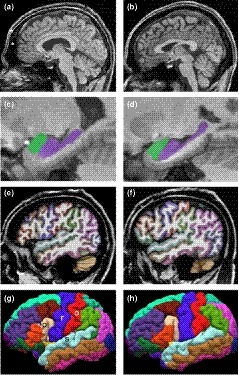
MRI findings. (a, c, e, g) The 26‐year‐old premutation carrier. (b, c, f, h) A 24‐year‐old male control. (a, b) A mid‐sagittal slice showing enlarged lateral ventricles and enlarged frontal subarachnoid spaces in the carrier (*). (c, d) Disproportionally enlarged hippocampal head the length of which is normally 35% of the whole length (Hackert et al., 2002).^23^ Hippocampus, purple; amygdala, lime. (e, f) A sagittal slice of the left hemisphere showing more complex folding in the carrier at the junction of the frontal, parietal, and temporal lobes. (g, h). A lateral view of the reconstructed cortex of the left hemisphere showing more complex folding in the pars opercularis (p, light brown, LGI = 4.49, *Z* = 2.38, 1.73%), precentral gyrus (r, purple, LGI = 3.79, *Z* = 4.32, 0.0008%), postcentral gyrus (o, red, LGI = 3.81, *Z* = 3.81, 0.007%), superior temporal gyrus (s, cyan, LGI = 4.32, *Z* = 2.58, 0.49%), and transverse temporal cortex (*, gray, LGI = 5.22, *Z* = 4.00, 0.0032%). Additional areas showing increased LGI are: left entorhinal cortex (LGI = 2.54, *Z* = 3.14, 0.085%), right postcentral gyrus (LGI = 3.72, *Z* = 2.28, *p* = .023), right superior temporal gyrus (LGI = 4.40, *Z* = 5.00, <0.0001%), and right transverse temporal gyrus (LGI = 5.07, *Z* = 2.66, 0.39%)
